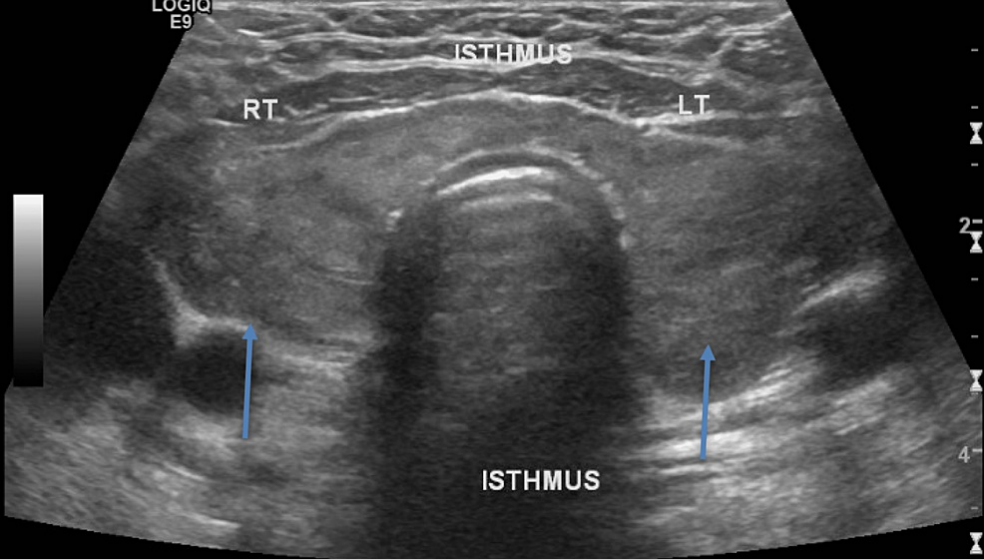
Ultrasound of the thyroid and parathyroid showing right and left thyroid lobes along with isthmus Of note is the heterogeneous thyroid with a nodule on the right as well as left sides (arrows).
